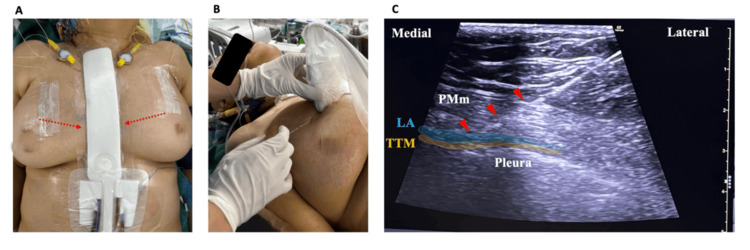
Deep parasternal intercostal plane block (DPIPB) procedure and catheter placement. (A) The red dotted arrows indicate the direction of catheter placement. (B) Linear ultrasound probe-guided placement and needle direction for DPIPB. (C) Ultrasound image showing the pectoralis major muscle (PMm), local anesthetic (LA), transversus thoracis muscle (TTM), and pleura. The red arrowheads indicate the needle trajectory.
